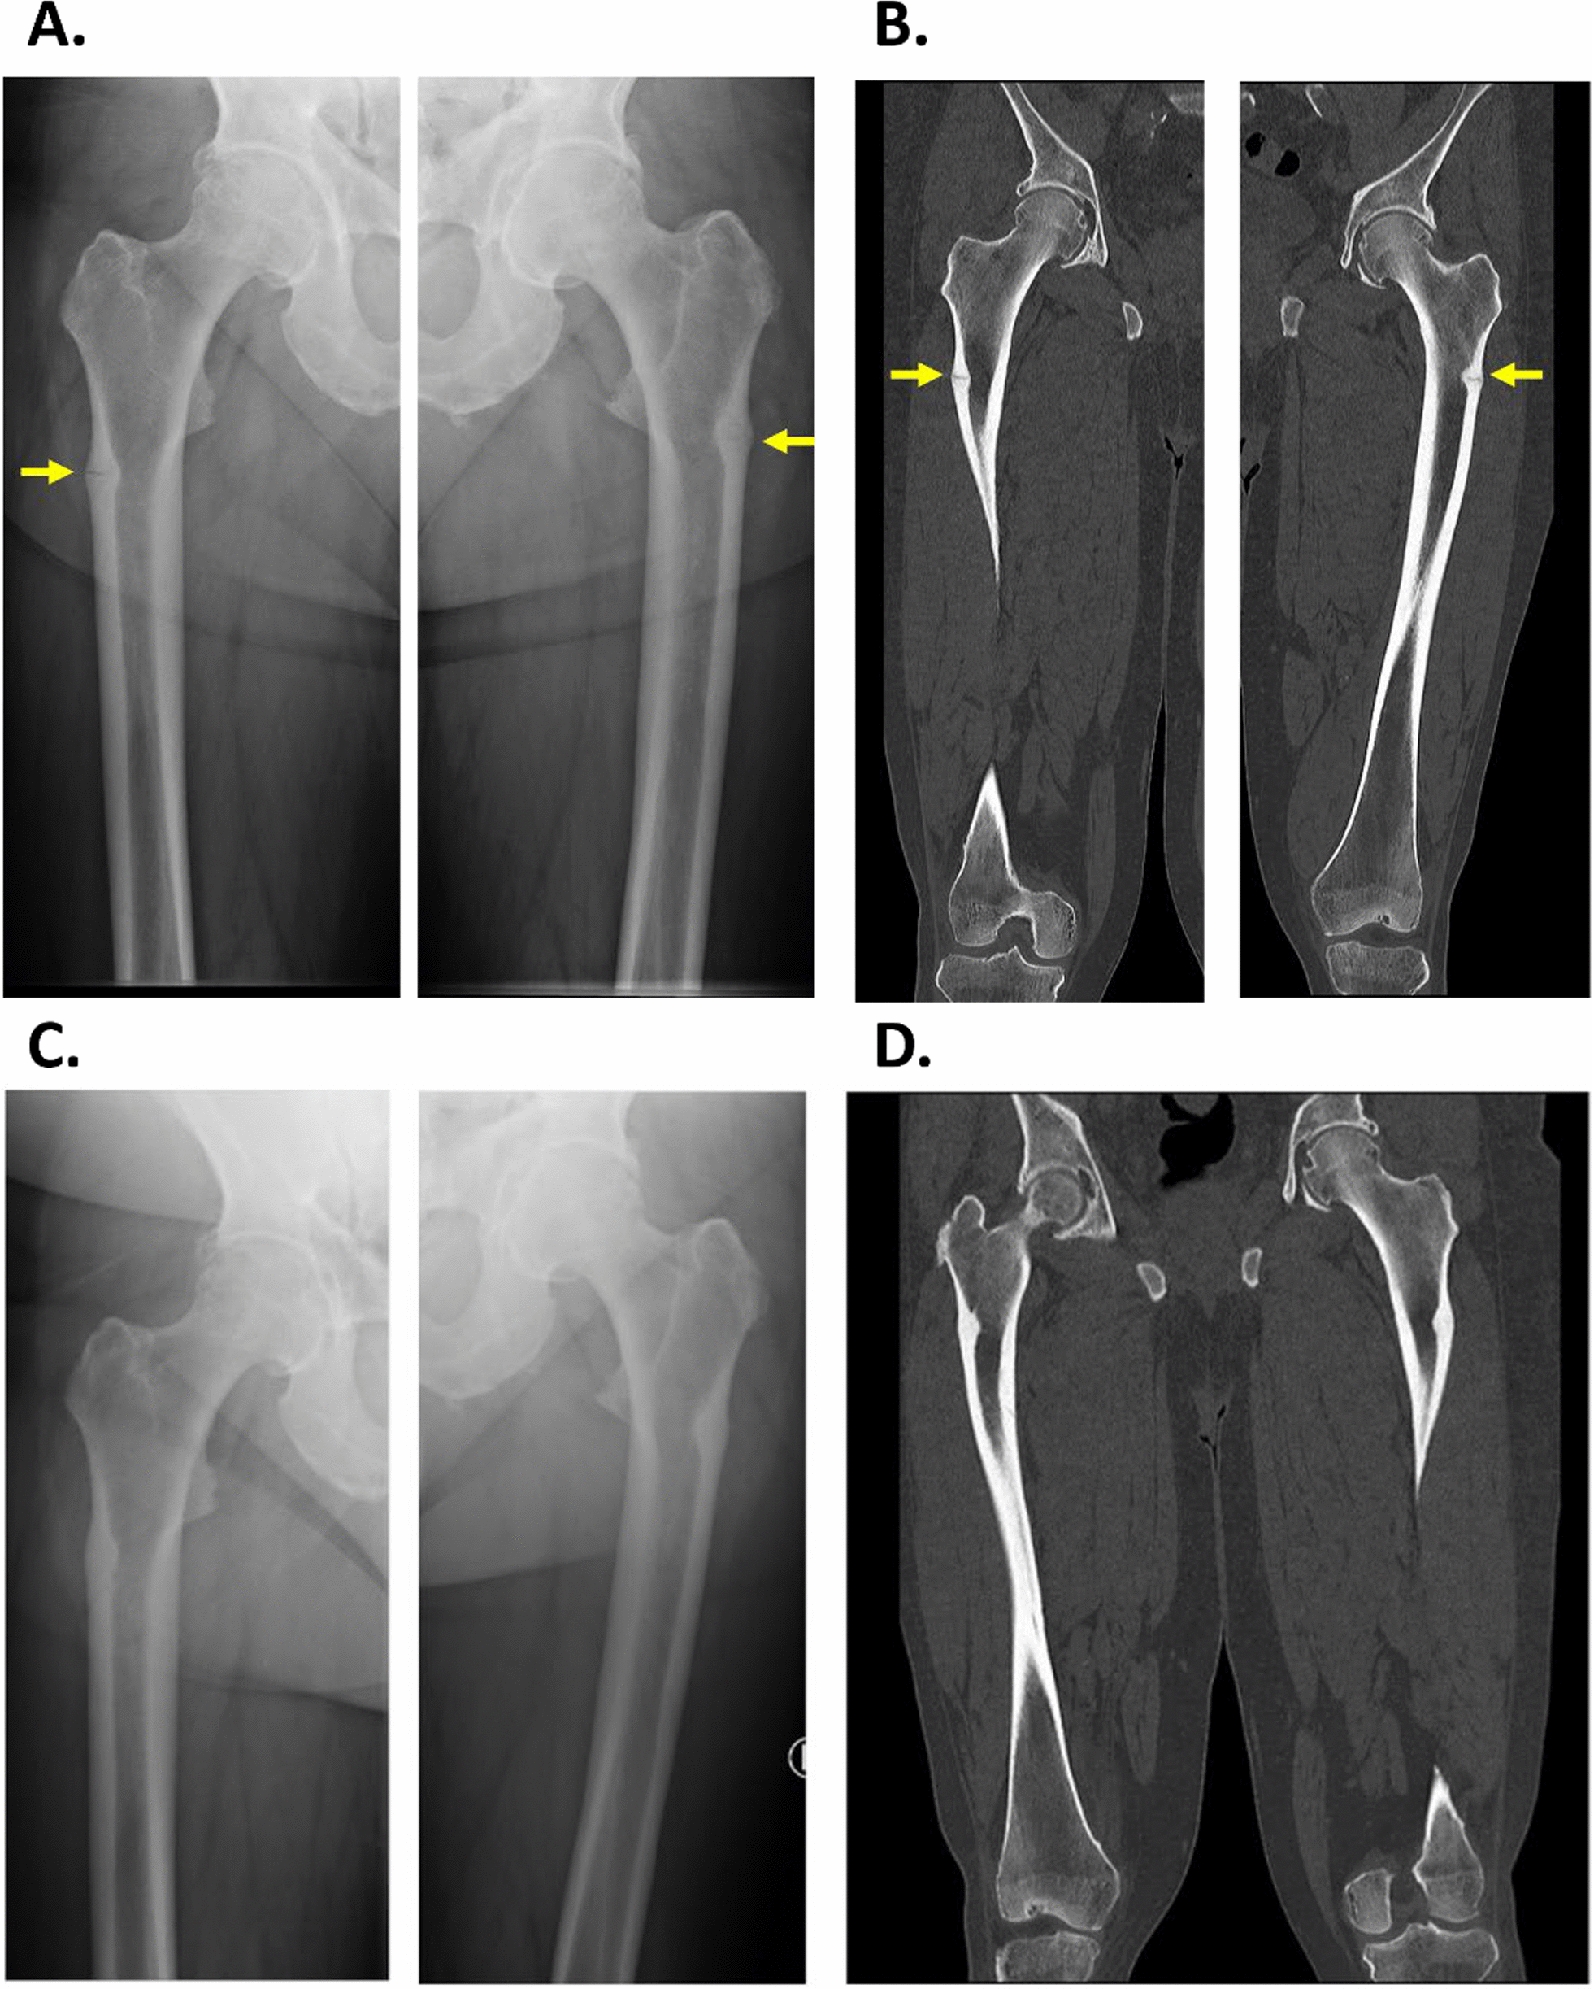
Results of X-ray and computed tomography scan controls. X-ray (A) and computed tomography scan (B) performed before treatment with teriparatide showed pseudofractures on the lateral aspects of the proximal femora bilaterally (yellow arrows). X-ray (C) and computed tomography scan (D) performed 17 months after treatment showed complete healing of both femoral pseudofractures
